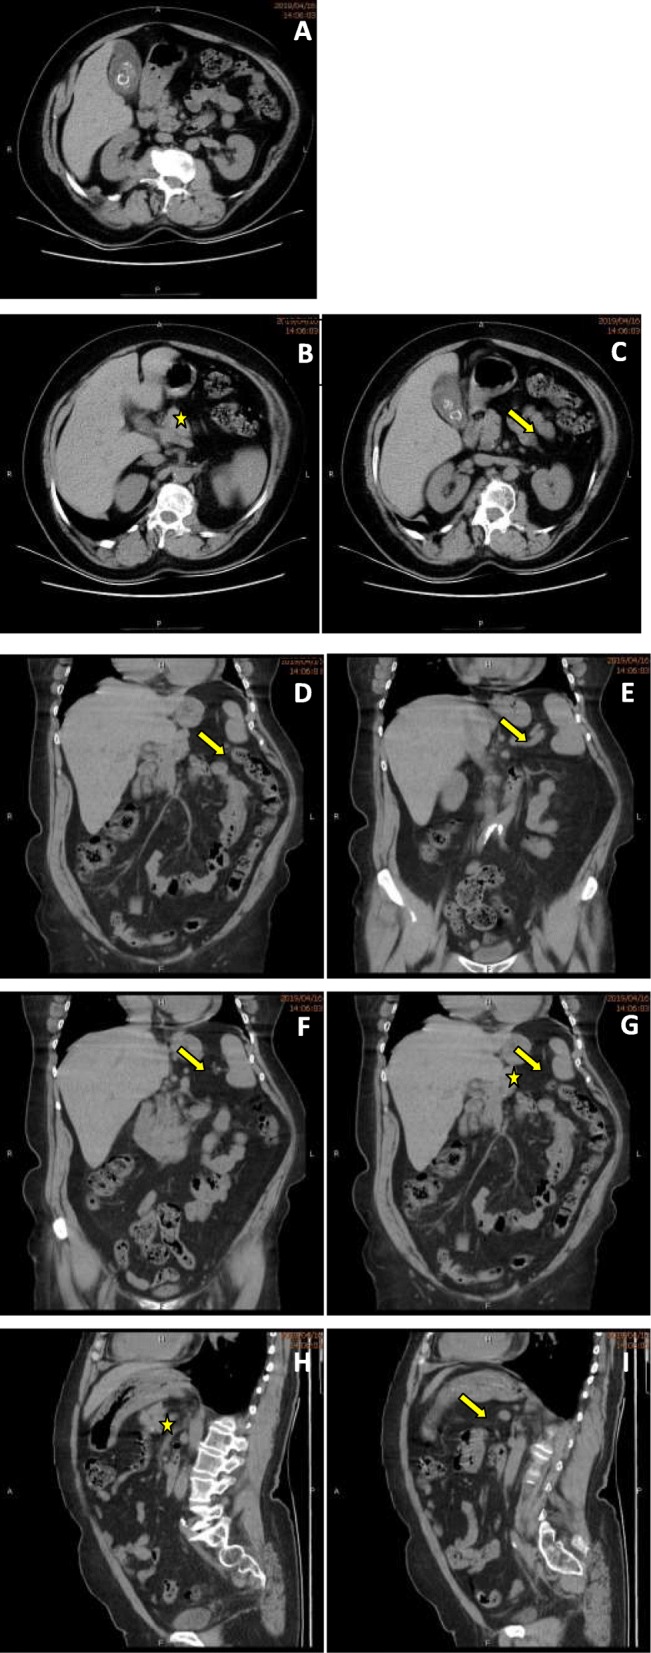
a Abdominal CT image shows the stones in the gallbladder and the inflamed gallbladder. b-i Abdominal CT image including coronal reconstruction (d-f) and sagittal reconstruction (h and i) image shows the head of the pancreas without the body and tail. The *star* shows the pancreas head, the *arrow* indicates the area of the body and tail of the pancreas and now only the retinal tissue and the blood vessels of the spleen is there
